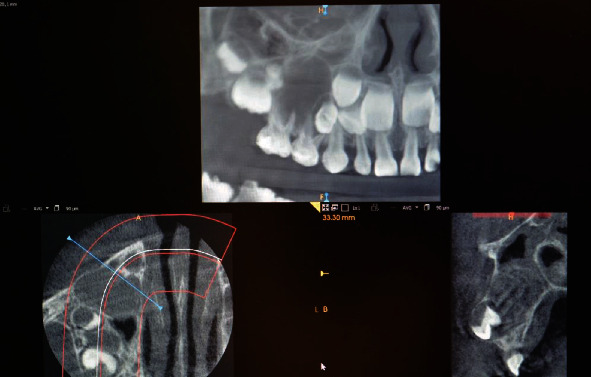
CBCT scans (GXDP-800, OP300-1, Gendex Dental Systems, 2017, Medical University of Lodz, Poland. Software: InVivoDentalViewer 5.1, Anatomage, Milan, Italy).
